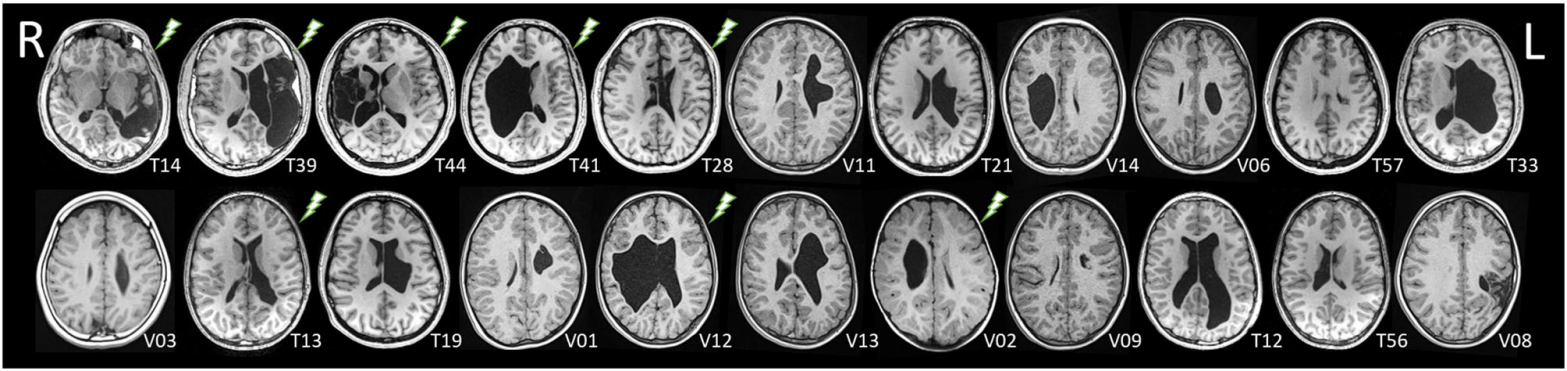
Axial slices of the T1-weighted datasets of the 22 patients with MRI data available, sorted by TONI scores (as in Table 1). No MRI was available in patient V04 due to dental braces. A “lightning” symbol marks patients with epilepsies. Radiological orientation, R, right; L, left.
